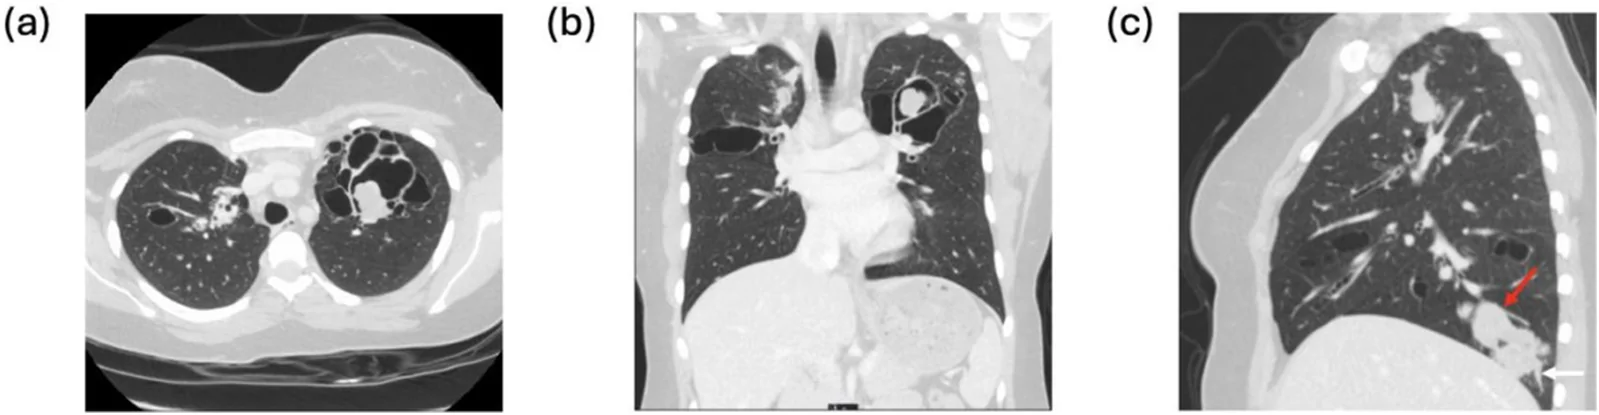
CT findings in a patient with an aspergilloma and concomitant allergic bronchopulmonary aspergillosis. (a) Axial and (b) coronal reconstruction showing an aspergilloma within a cystic cavity in the left upper lobe, (c) Sagittal view demonstrating hyperattenuating mucoid impaction within a dilated bronchus in the right lower lobe (red arrow), with adjacent mild tree-in-bud nodularity (white arrow).
